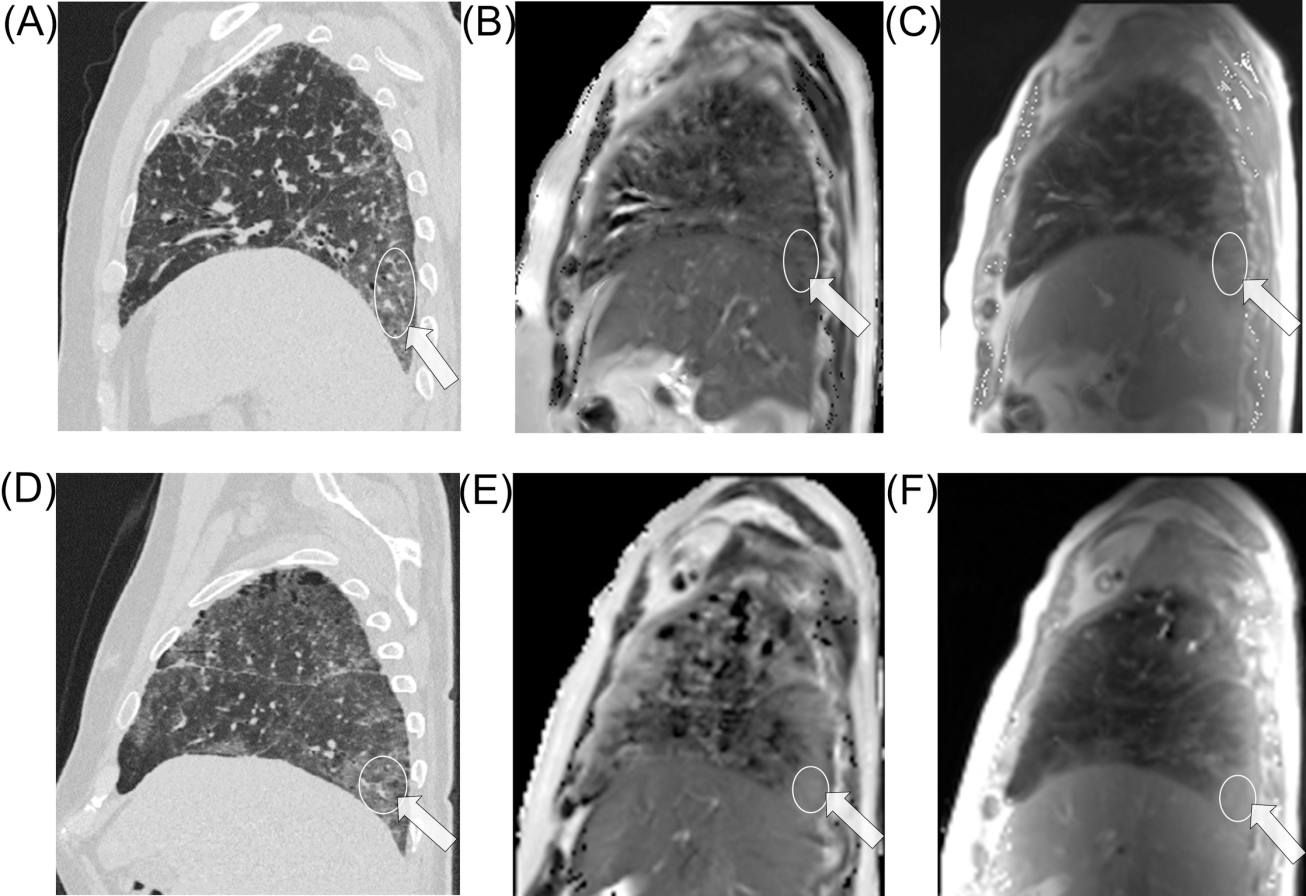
A 59 year old male patient with stable NSIP: image A is the sagittal reformat CT reference image, with the arrow indicating the region of interest, followed by the T2 map, image B and PD intensity map, image C. Below is the case of a 65 year old male patient with NSIP and suspected inflammatory activity and his corresponding images, D to F. Both cases show similar CT morphology and inhomogeneous T2 relaxation and PD of the lung; there is higher T2 relaxation and PD intensity on both type of maps in the active disease case. Exemplary ROI placement in relevant pathological areas is demonstrated.
